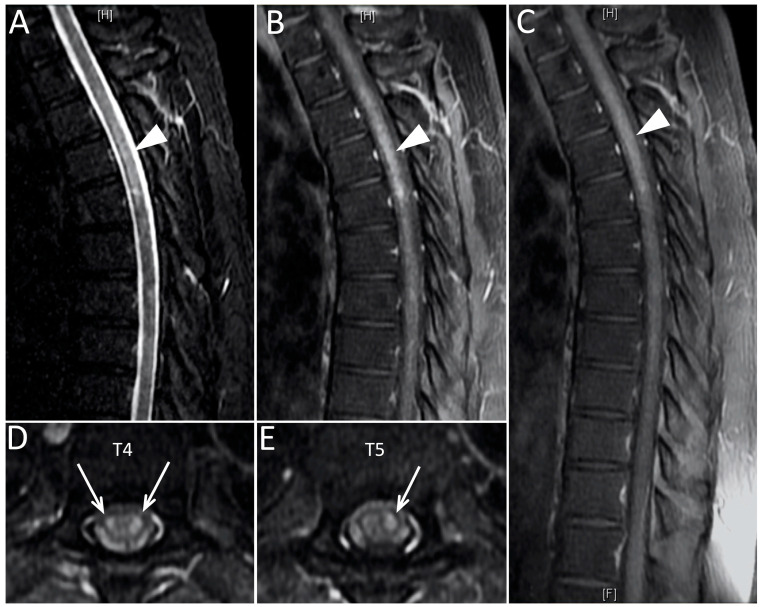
The MRI of the thoracic spine on the T2-weighted short tau inversion recovery scan (A) showed a hyperintense lesion on T1-T6 (indicated by arrowheads). The lesion was enhanced in the early-phase contrast-enhanced T1-weighted fast spin echo scan (B) and post-contrast T1-weighted fast spin echo scan (C). The axial view of the post-contrast T1-weighted fast spin echo scan over T4 (D) and T5 (E) revealed the enhanced lesion in the spinal cord (indicated by arrows).
